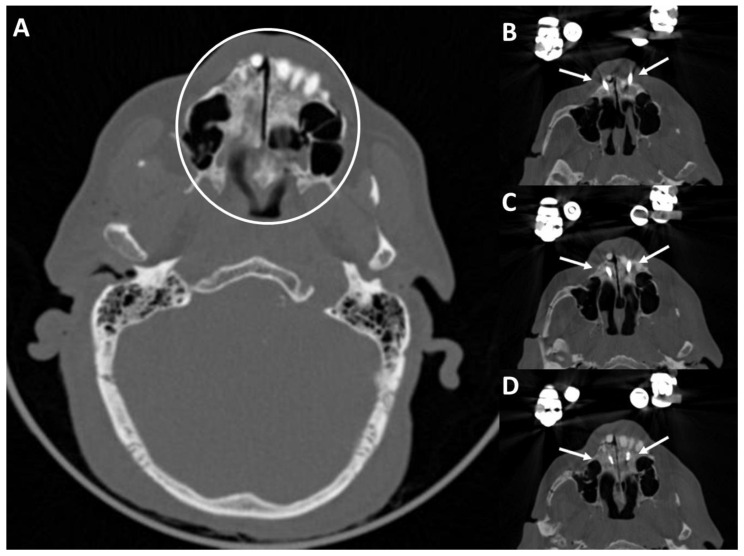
CT scans of the fractured skull (A) and palatal split (white circle). (B–D) Reduced palatal split with two medial palatal pins (white arrows).
